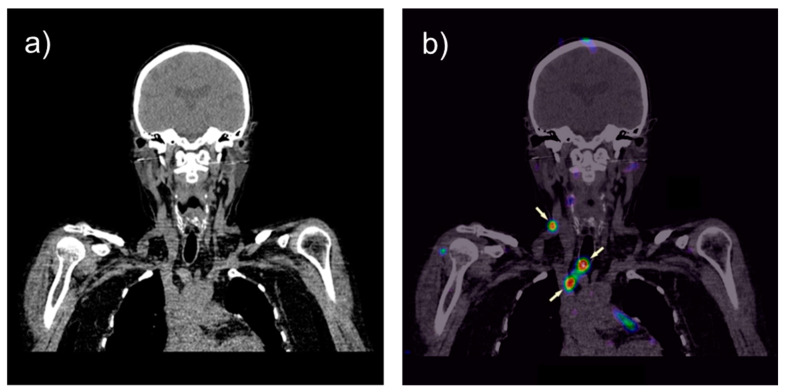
CT (a) and fused [^111^In]In-CP04 SPECT/CT (b) images in coronar orientation. Neck lymph node metastases and tumor infiltration around the tracheotomy tube (arrows) in a female patient with MTC are visible; images performed 24 h after injection of 210 MBq (50 µg peptide). Scan was performed within GRAN-T-MTC (ERA-NET on Translational Cancer Research (TRANSCAN), First Joint Transnational Call (JTC 2011) on: “Validation of biomarkers for personalised cancer medicine”, funded by the European Commission under the Seventh Framework Programme (FP7) with the following national co-found institutions: Ministry of Health (MoH), Italy, National Centre for Research and Development (NCBiR), Poland, Federal Ministry of Education and Research (BMBF), Germany, Austrian Science Fund (FWF, Project No. I1224-B19), Austria, Ministry of Higher Education, Science and Technology (MHEST), Slovenia, and General Secretariat for Research and Technology, Ministry of Education, Life Long Learning and Religious Affairs (GSRT), Greece.
